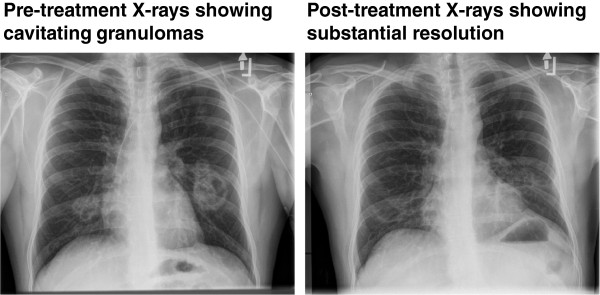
Chest radiographs demonstrating cavitation granulomas in a patient with GPA before and after treatment.
